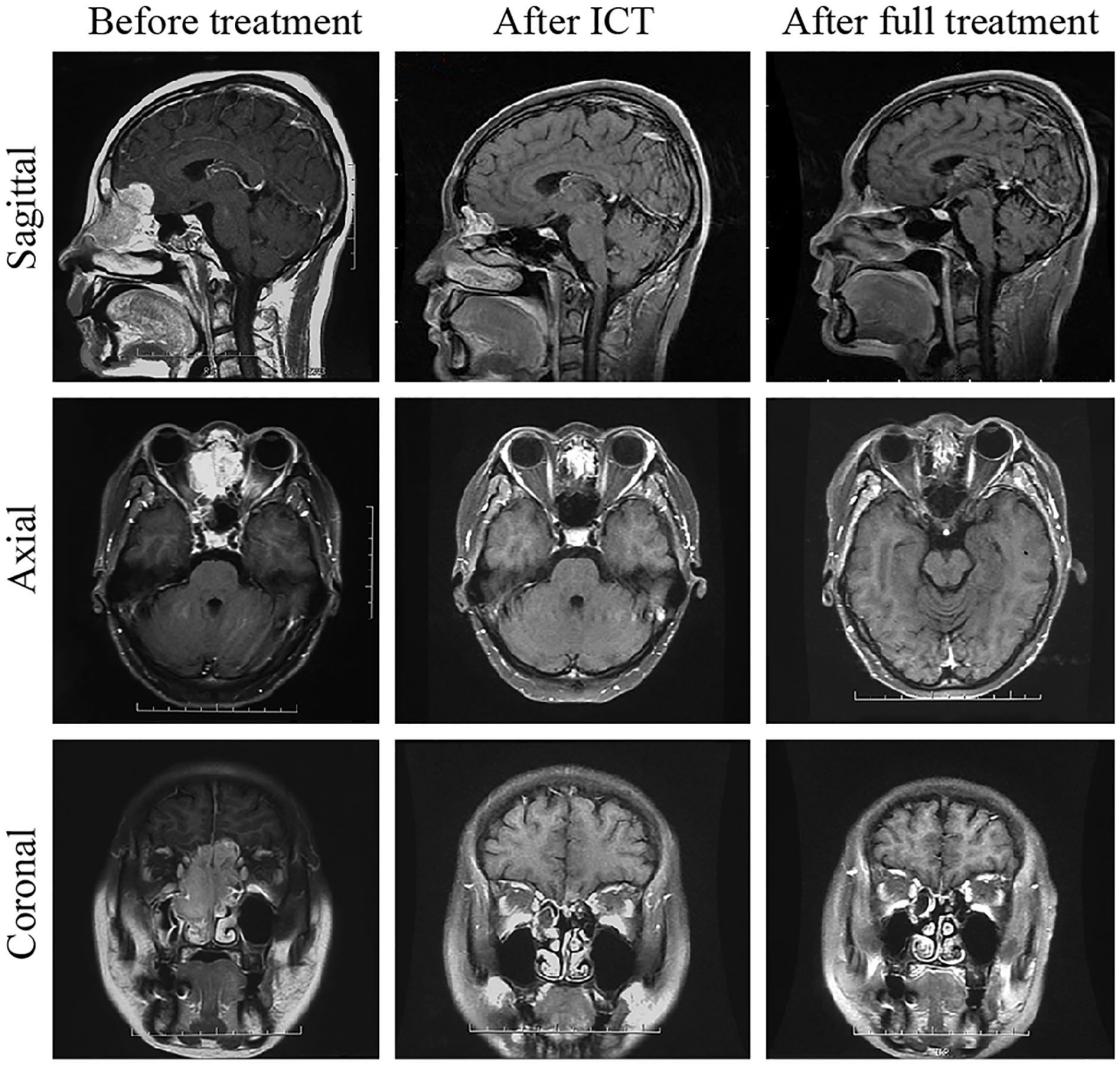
Comparison of MRI results before and after treatment of one patient with neuroendocrine carcinoma. One month after the treatment, the tumor had completely subsided. The patient was followed up for 54 months and remained free of disease.
